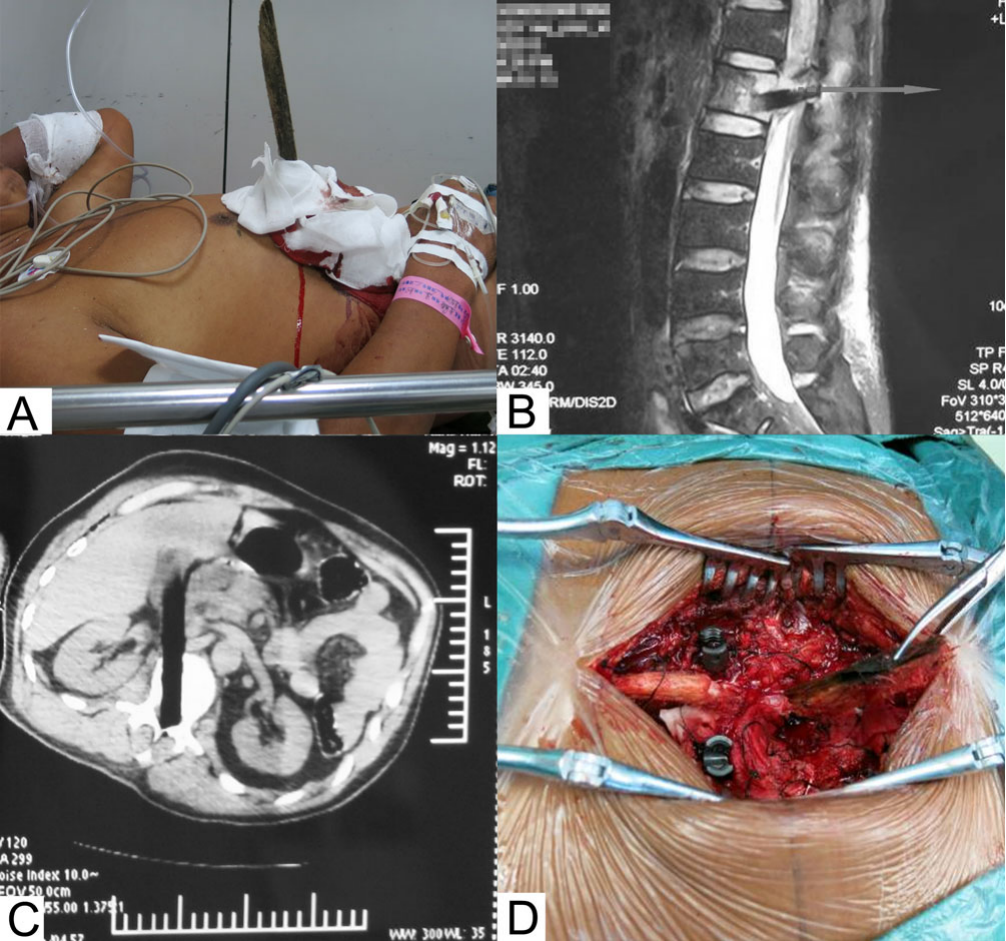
Case 3 (60‐year‐old male). (A) the patient presented with a penetrating injury from a wooden stick; (B) sagittal MRI demonstrated a foreign body penetrating the L_1_ vertebra and spinal canal; (C) coronal computed tomography revealed a foreign body extending through the liver, inferior vena cava, kidney and L_1_ vertebra and (D) laminectomy and internal fixation with lateral mass screws
